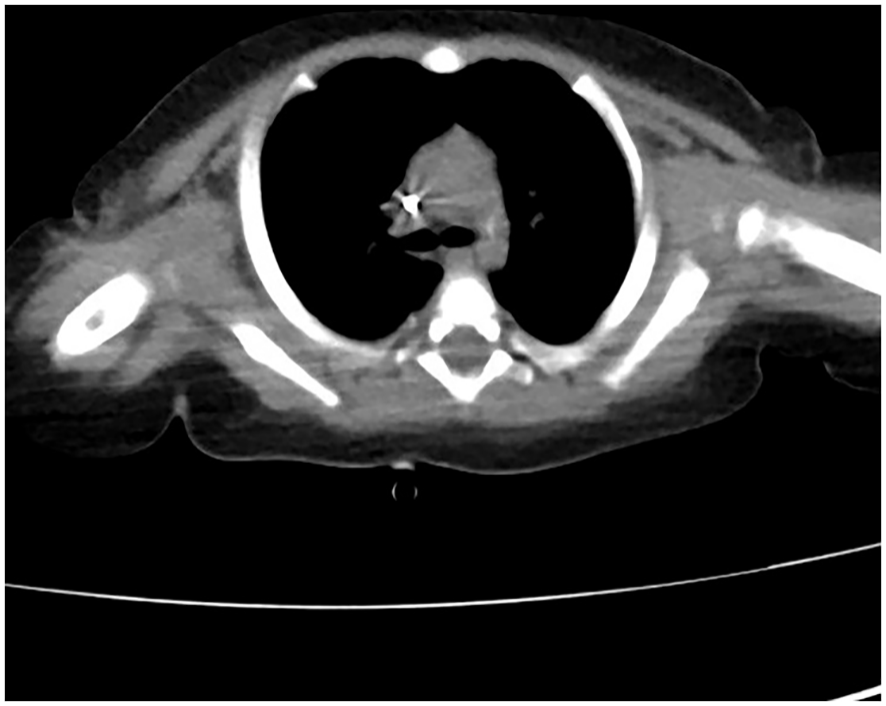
The chest CT revealed the absence of a thymus.
